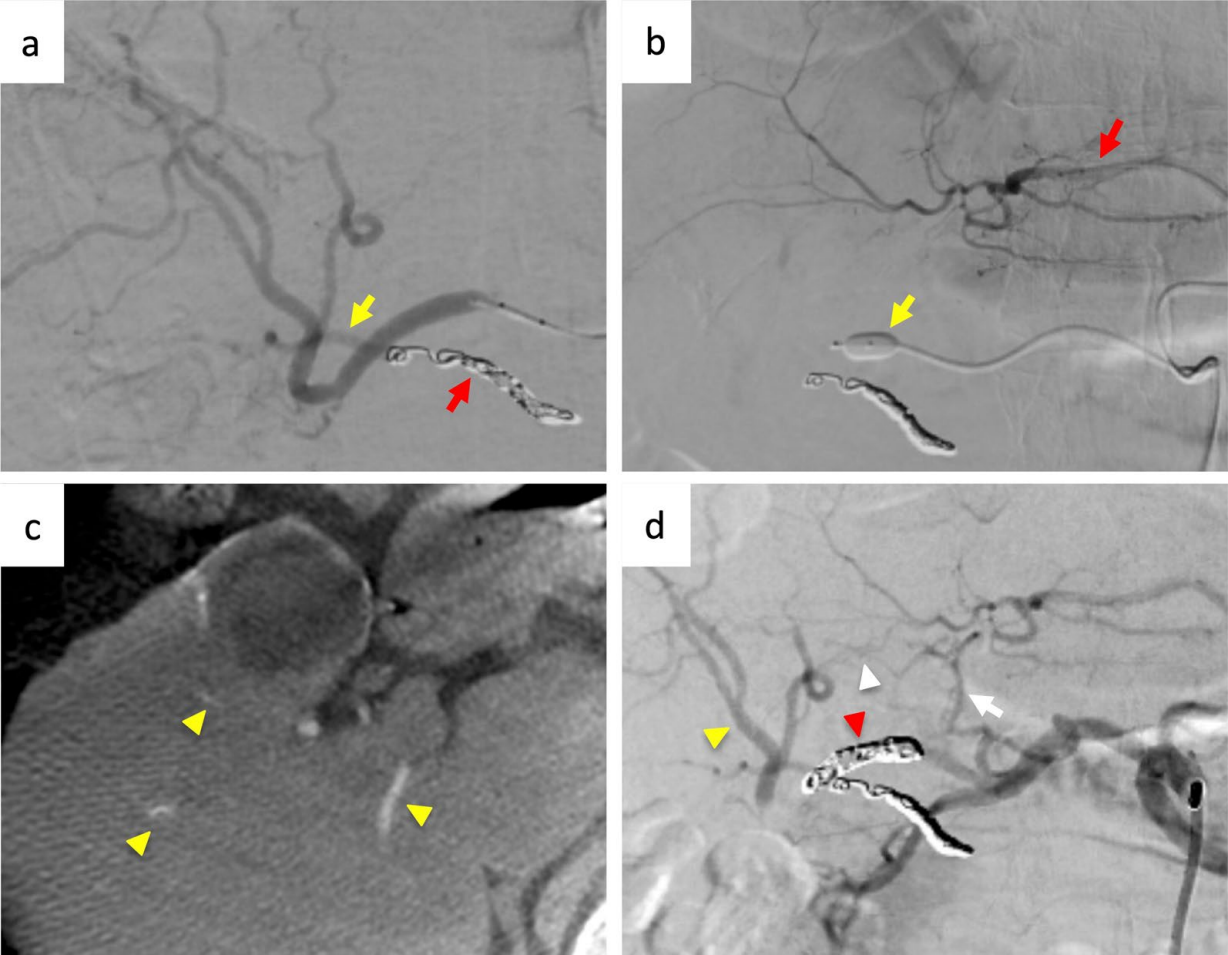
Preoperative angiography and hepatic artery embolization. a Selective angiography from the RHA after an embolization of aRHA (red arrow) showed the intrahepatic artery comprised of aRHA was compensated by RHA (yellow arrow). b Selective angiography of the rLHA after temporally occlusion of RHA using micro balloon catheter, which did not detect the collateral artery from LHA to RHA. c Cone-beam CT of (b) detected RHA (yellow arrowhead) fed by LHA. d Selective angiography of the celiac artery after an embolization of RHA (red arrowhead) detected LHA and the middle hepatic artery (white arrow) and collateral artery (white arrowhead) and RHA (yellow arrowhead). *CT* computed tomography, *RHA* right hepatic artery, *aRHA* accessory right hepatic artery, *rLHA* replaced left hepatic artery
